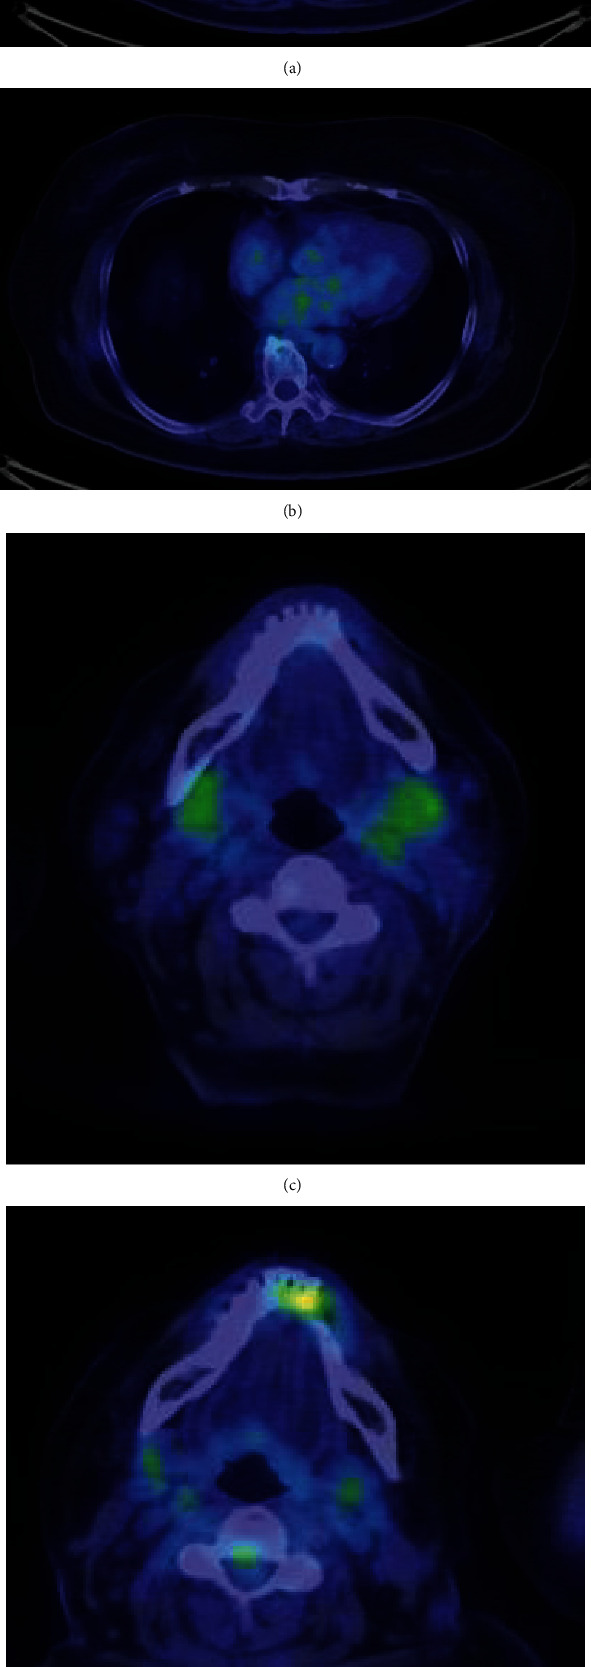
Positron emission tomography (PET)/CT images before and after steroid therapy. Abnormal ^18^F-FDG uptake was found in the pericardium (a) and submandibular gland (c) before treatment. After treatment, ^18^F-FDG uptake in the pericardium (b) and submandibular gland (d) is diminished.
